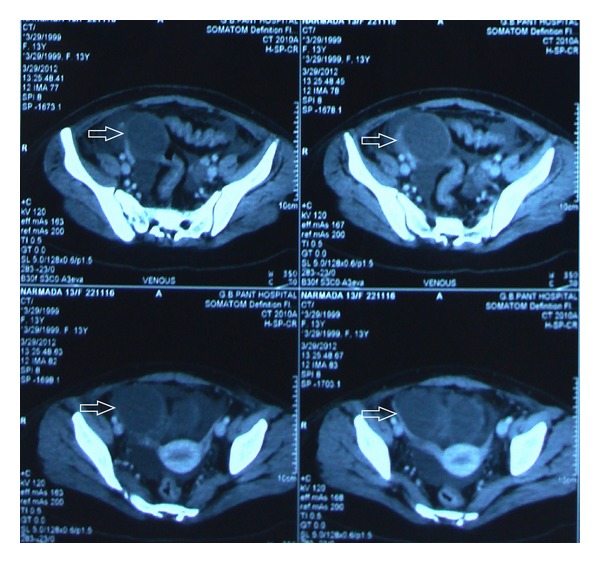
CT sections showing a hemorrhagic ovarian cyst.
